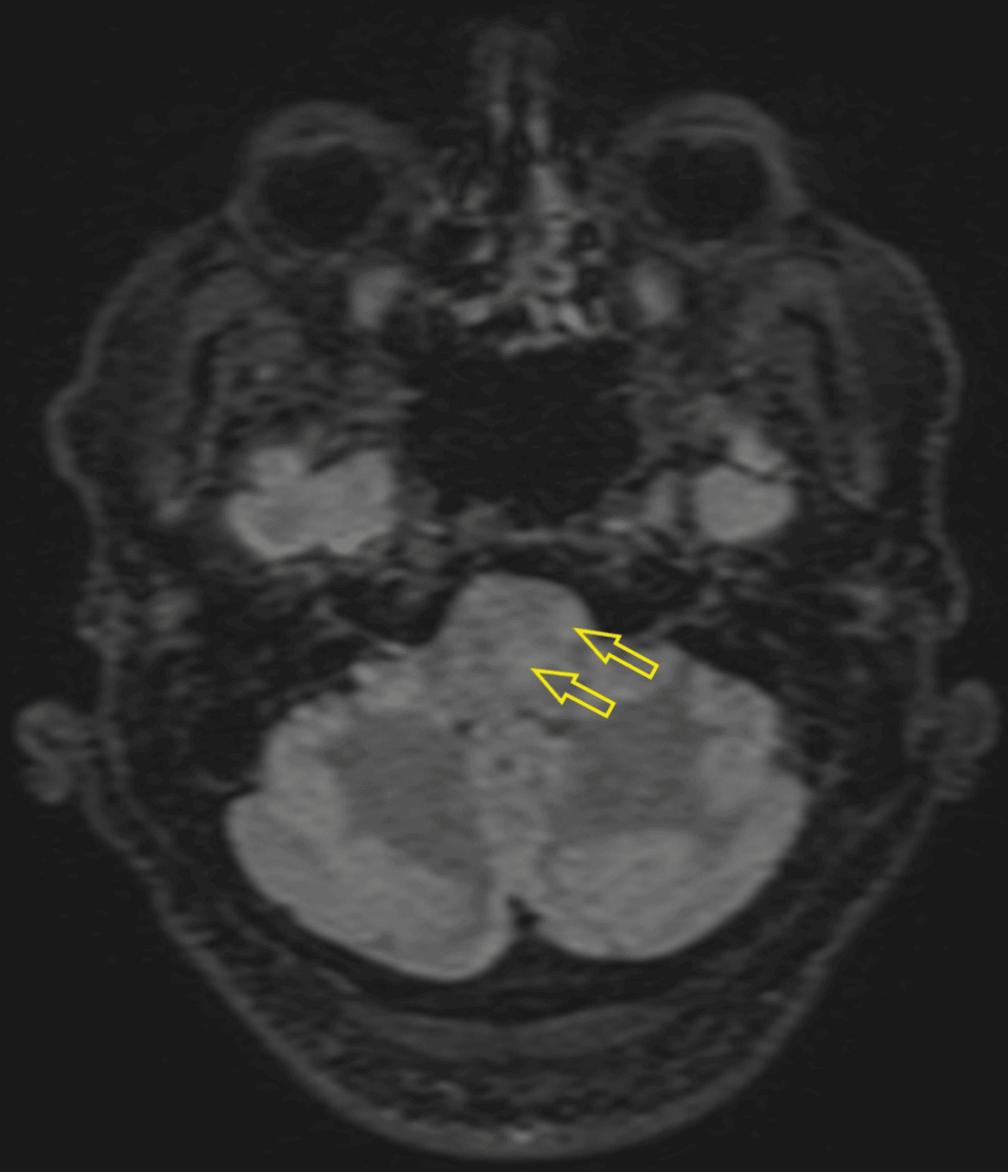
Axial fluid-attenuated inversion recovery (FLAIR) MRI demonstrating patchy hyperintense lesions in the central and left ventral pons.
